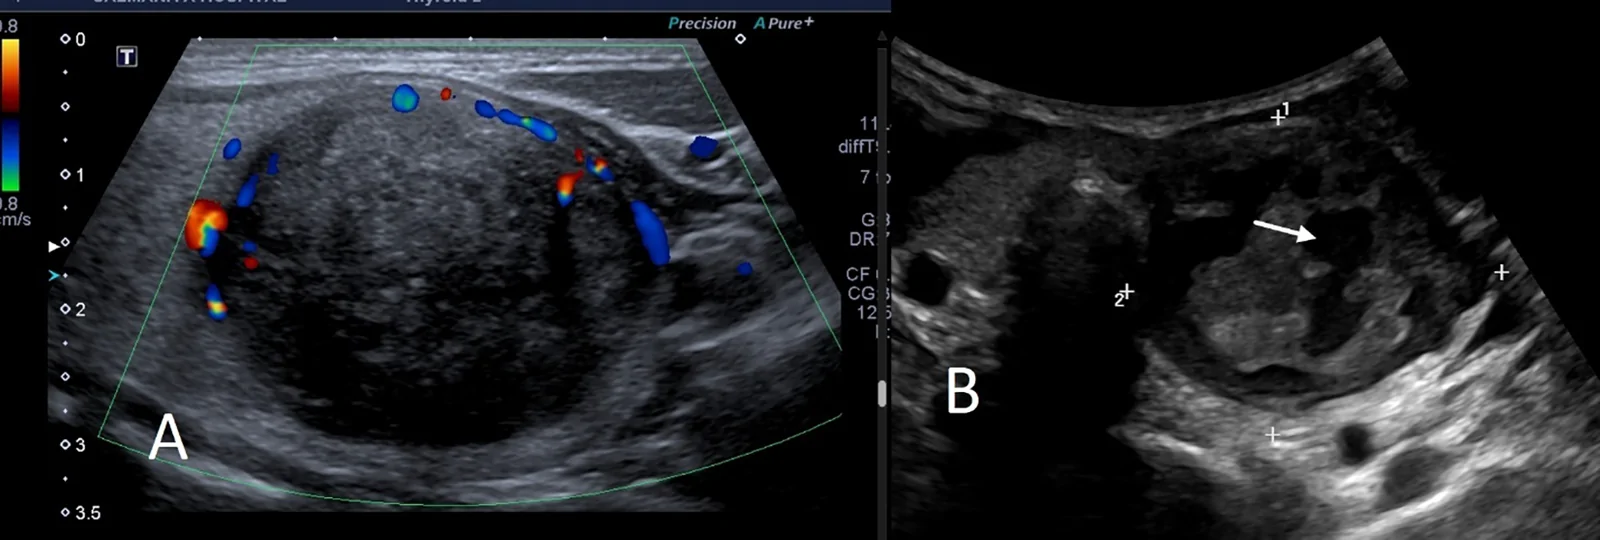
Thyroid ultrasound findings at initial presentation and follow-up. (A) Ultrasound examination performed at initial presentation in 2020 demonstrating a well-circumscribed hypoechoic solid lesion in the left thyroid lobe with mild peripheral vascularity on color Doppler imaging and no associated calcifications. (B) Follow-up ultrasound examination in 2025 showing a marked interval increase in lesion size, with a heterogeneous hypoechoic appearance and scattered cystic degenerative changes.
